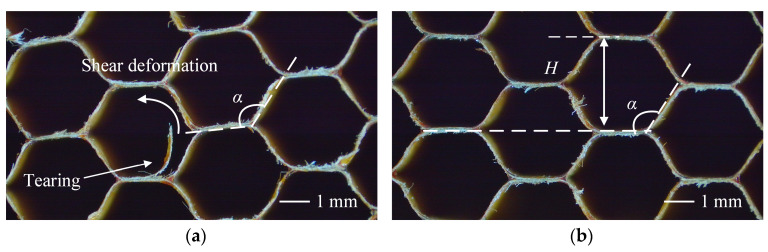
(a) Surface morphology without UV: feed rate and spindle speed at 3000 mm/min and 3000 r/min, respectively. (b) Surface morphology with UV: ultrasonic amplitude at 20 μm, spindle speed at 3000 r/min, and feed rate at 3000 mm/min.
